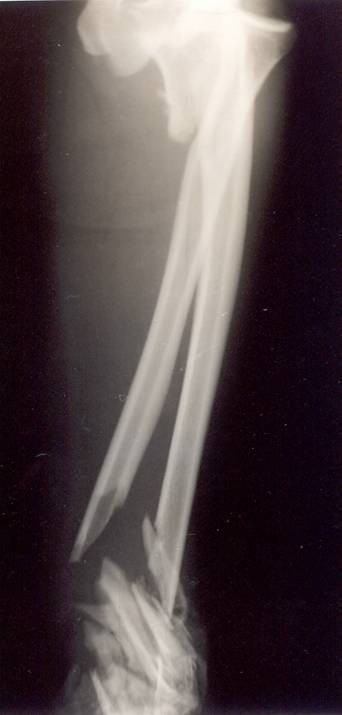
Radiographs of a 45-year-old man multiply injured who had an open complex injury of his left elbow and an ulnar nerve injury after a road traffic accident. Anteroposterior radiographs show a Monteggia fracture dislocation of the left upper arm with additional comminuted fractures of the distal end of both radius and ulna.
